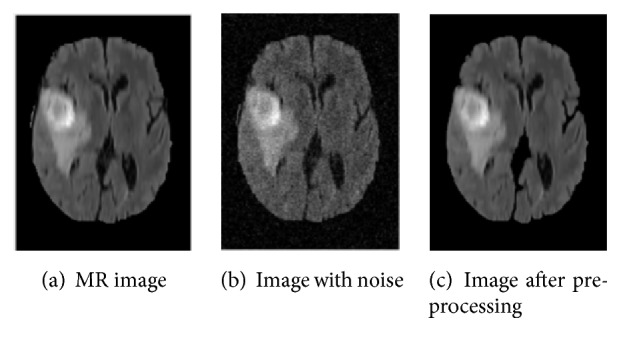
Example of adding Gaussian noise (variance, 0.02) to the MR image for denoising and the resulting image obtained after preprocessing.
